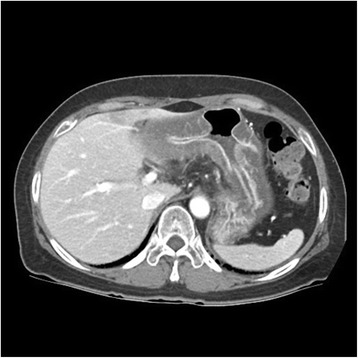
CT shows diffuse low density wall thickening of stomach
